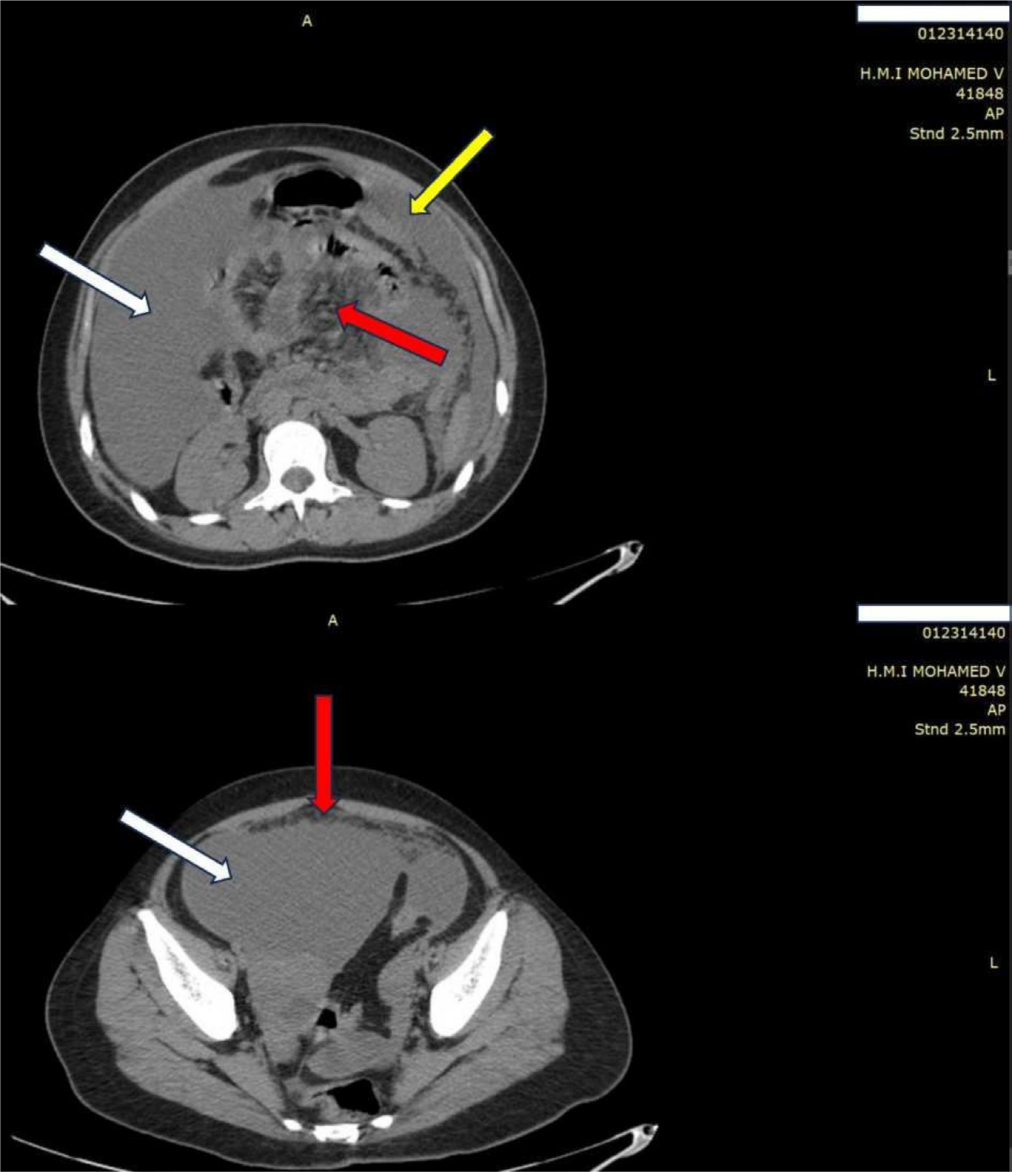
Abdominal computed tomography scan showing abundant partitioned ascites (white arrows) associated with peritoneal thickening (yellow arrow) and diffuse mesenteric infiltration (red arrows). A= Frontal view, L=Lateral view.
